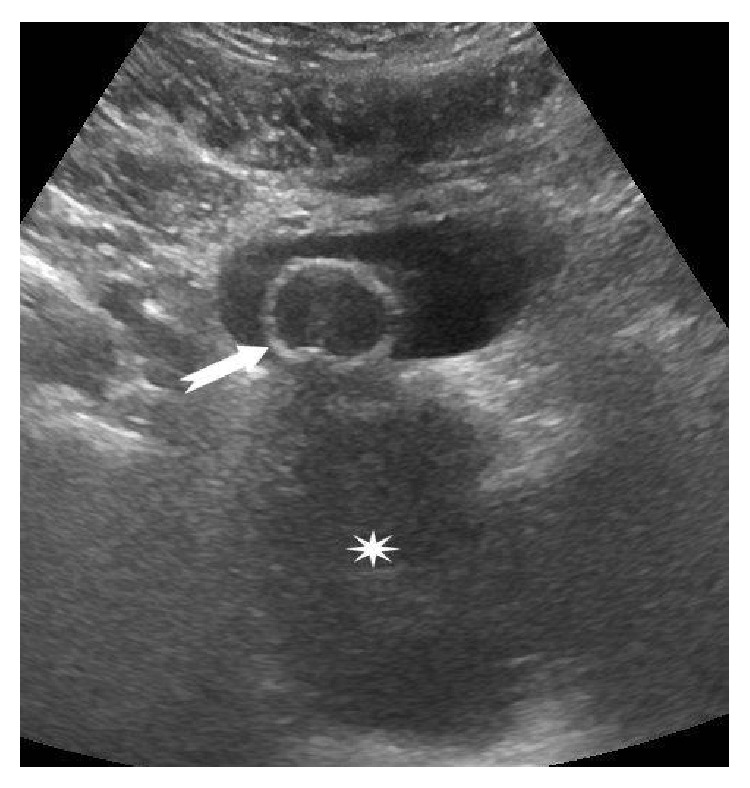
Pelvic ultrasound. A large hypoechoic mass (asterisk) is seen in the prostatic loggia. The arrow indicates the urinary catheter.
